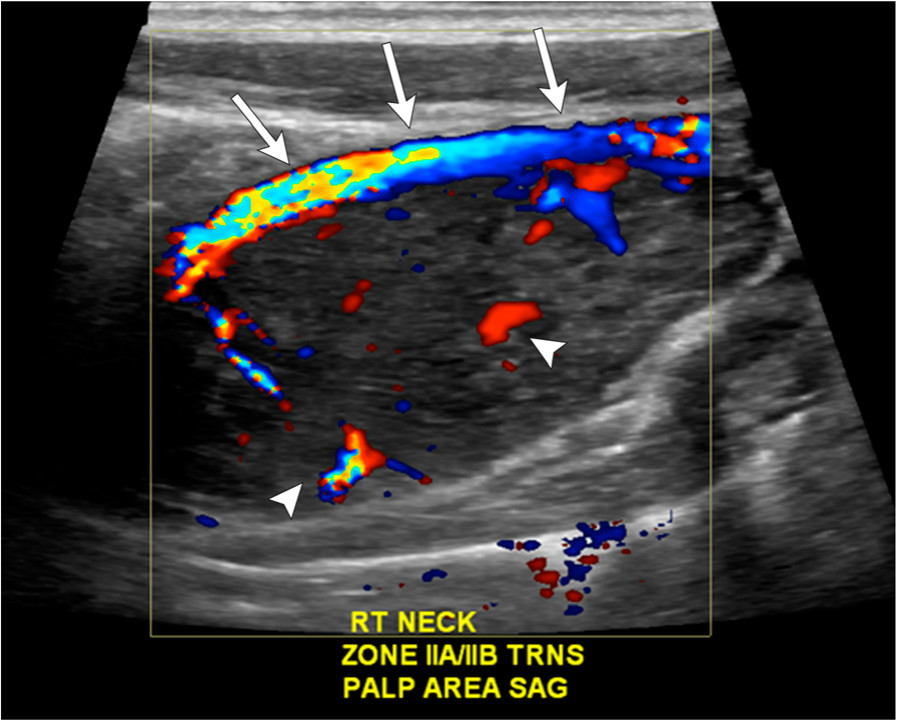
Color Doppler ultrasound image of the right cervical neck demonstrates a relatively hypoechoic, well-circumscribed mass with internal vascular flow (white arrows) in the right level II region. The mass splays the external carotid artery superficially (arrowheads)
